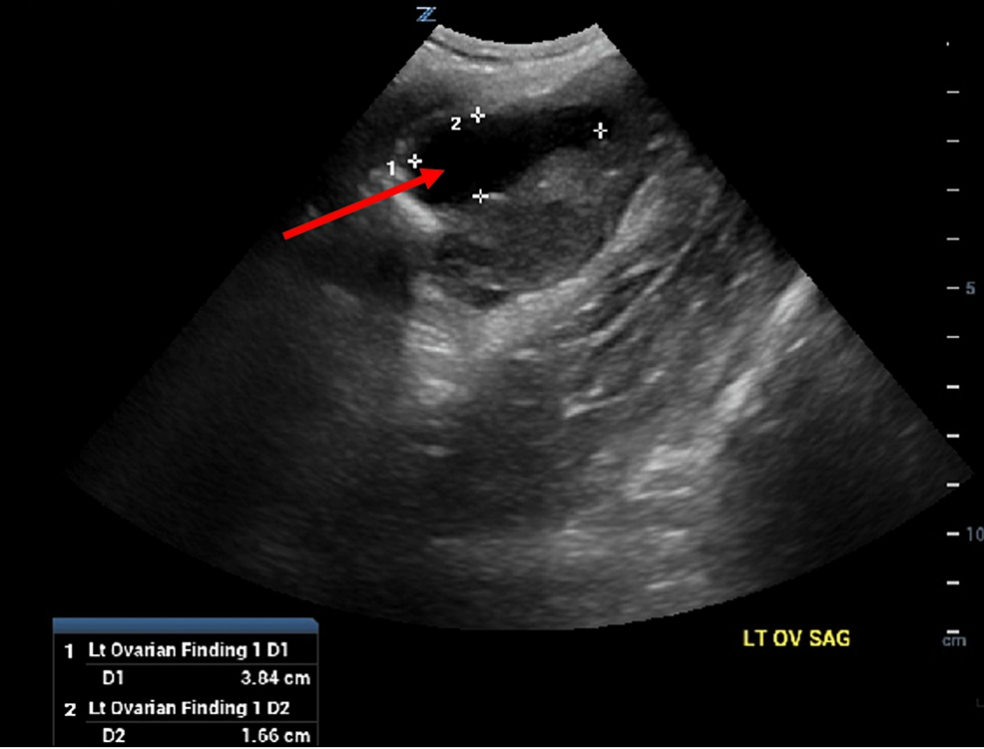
Complete pelvic ultrasound; view of the left adnexa.
